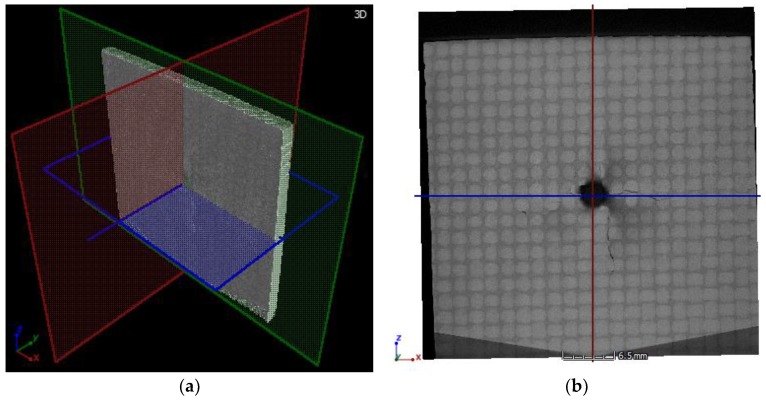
The X-ray computed tomography (CT) results: (a) a 3D view; (b) an exemplary front section.
